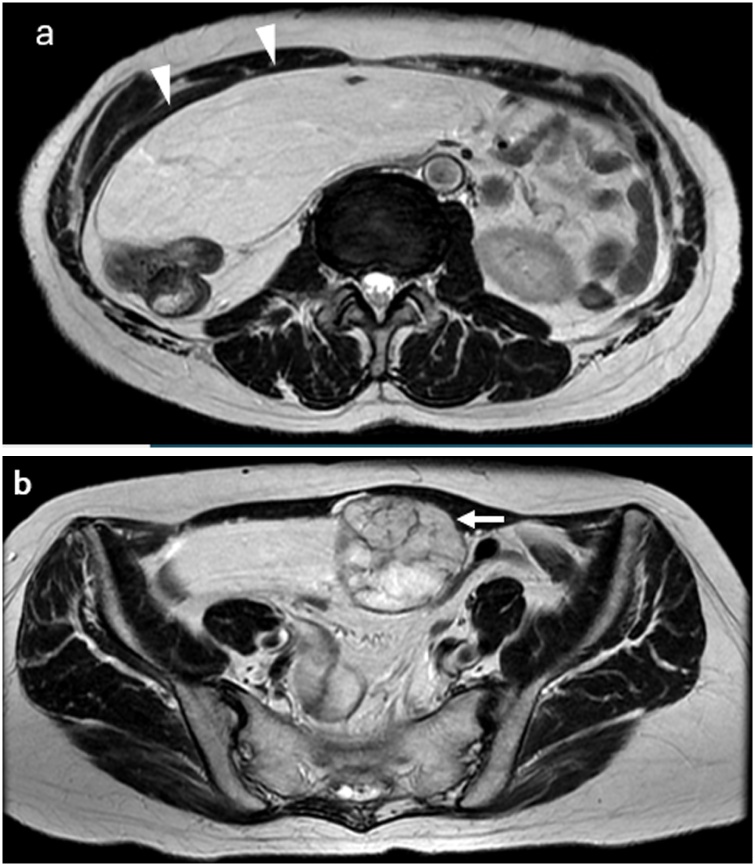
(a) T2-weighted MRI showed a large, well-defined, low-signal mass measuring 20 × 17 × 7 cm (arrowhead) in the abdomen. (b) The mass was adjacent to another abnormal region measuring 6 × 6 × 5 cm with septae and a capsule (arrow).
